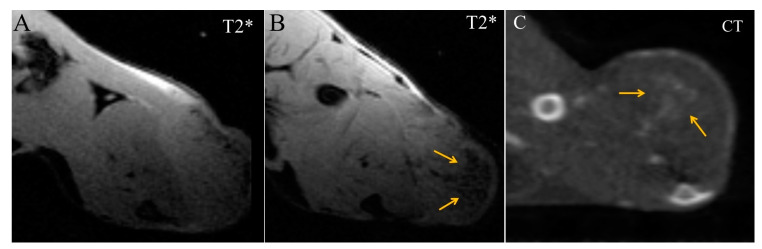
Transversal images of Ca755 mammary carcinoma. (A) native T2* MRI; (B) T2* MRI 16 min post injection of Fe_3_O_4_@Au; (C) CT image 22 min post injection of Fe_3_O_4_@Au. Arrows mark a triangular-shaped area of accumulation.
